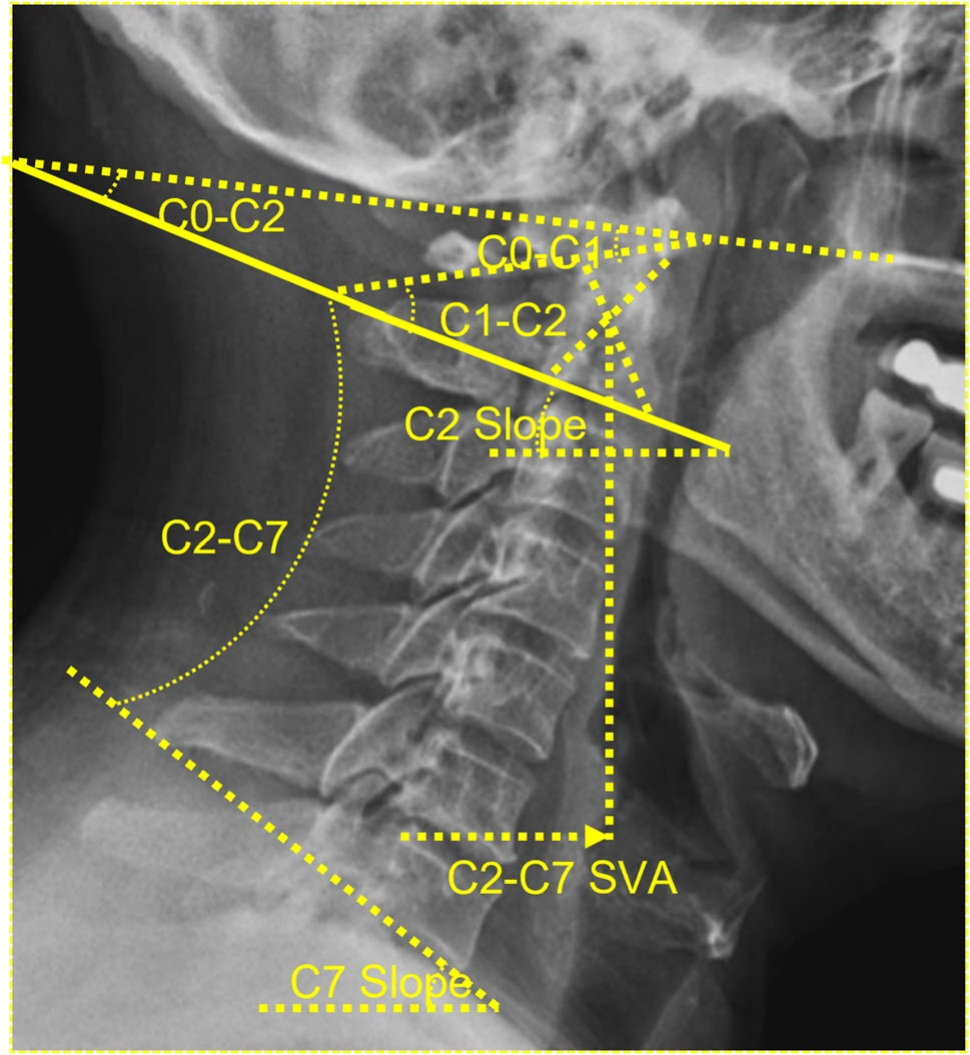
Measurement of radiographical parameters on X-ray examination
